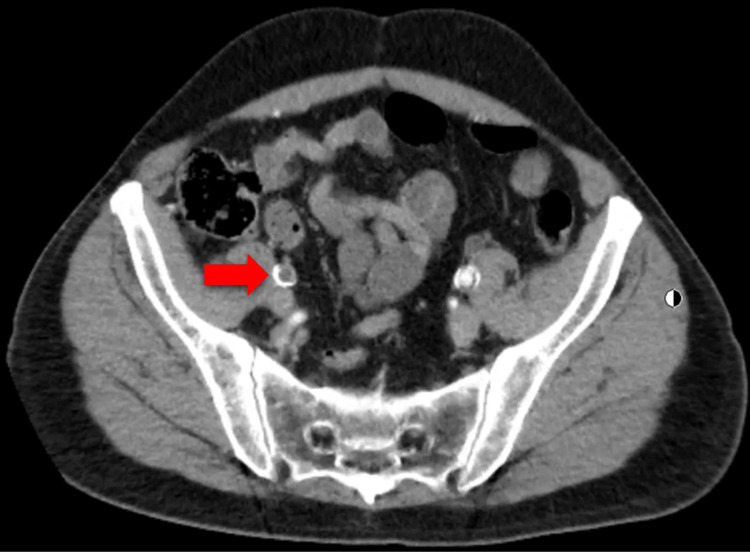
Abdominal pelvic computed tomography (CT) scan with contrast showing right external iliac artery occlusion. Red arrow, right external iliac artery occlusion.
